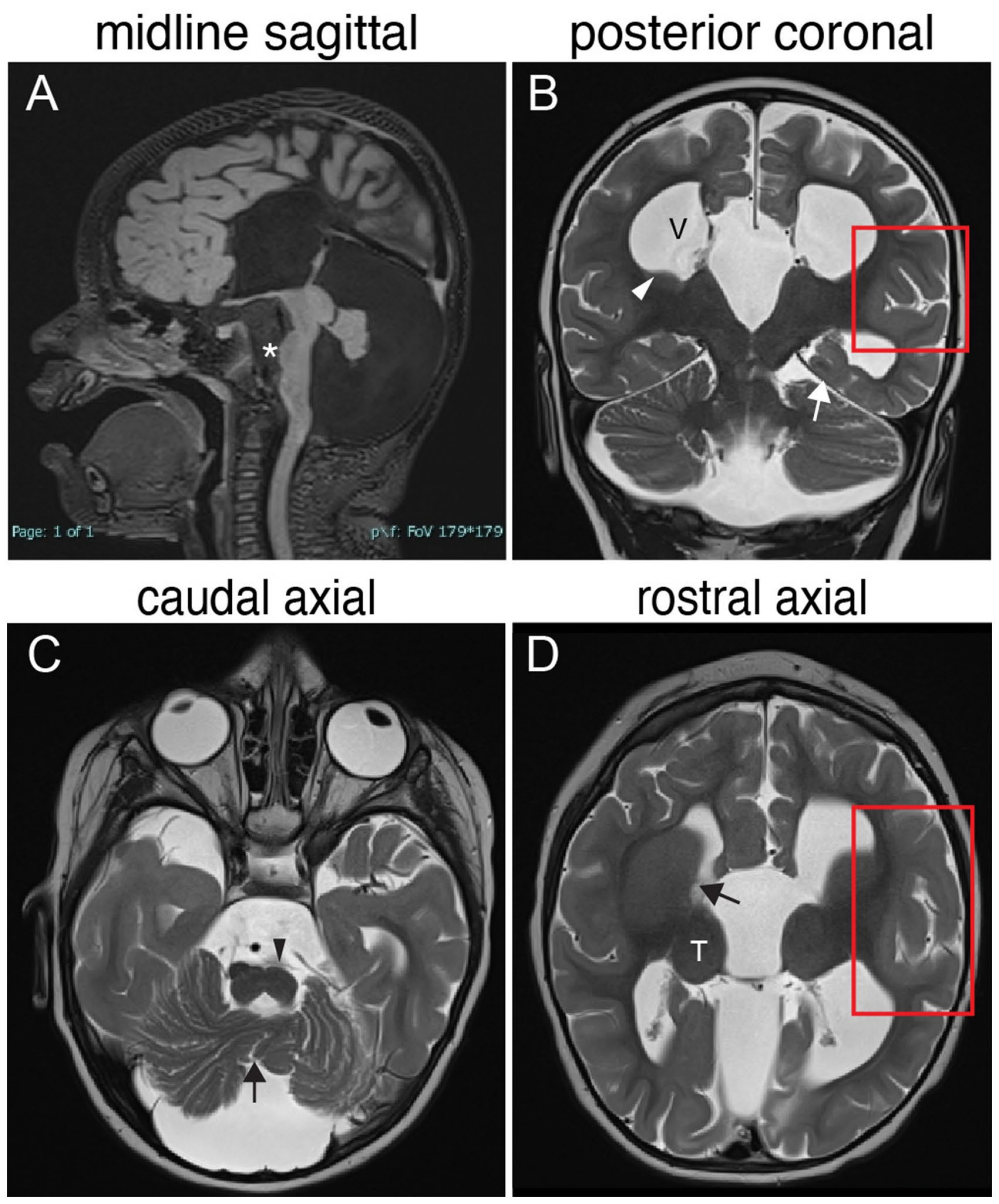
TUBB3-MCD brain malformations in individual harboring a TUBB3 T178M substitution. 17-month-old born at term presented with microcephaly, global developmental delay, axial hypotonia, appendicular hypertonia, and infantile spasms and underwent MR imaging. (A) Midline sagittal MR image: agenesis of the corpus callosum and anterior commissure, dysmorphic dilated ventricles, uplifted hypoplastic cerebellar vermis with enlarged CSF spaces, effaced aqueduct, malformed brainstem with flattened pons (asterisk). (B) Posterior coronal MR image: enlarged ventricles (V), bilateral hypoplasia of the caudate body and tail (white arrowhead), abnormal under-rotated left hippocampus (white arrow), abnormally oriented cerebellar folia, and thickened malformed cortex in the left Sylvian fissure (red boxed region). (C) Caudal axial MR image: hypoplastic malformed cerebellar vermis (black arrow) and hemispheres, flatted left pyramidal track (black arrowhead) and enlarged CFS spaces. (D) Rostral axial MR image: malformation of the basal ganglia with fusion of the caudate and putamen with no visible anterior limb of the internal capsule (black arrow), rounded globular-appearing thalami (T), ventricular enlargement, and thickened malformed cortex around the left Sylvian fissure (red boxed region).
